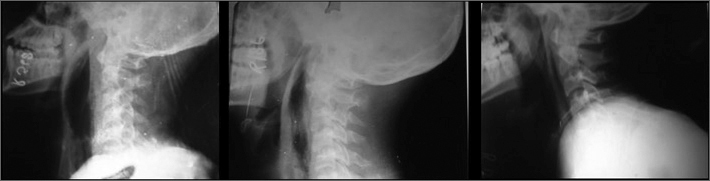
sequential lateral neck x-rays showing resolution of the abscess
